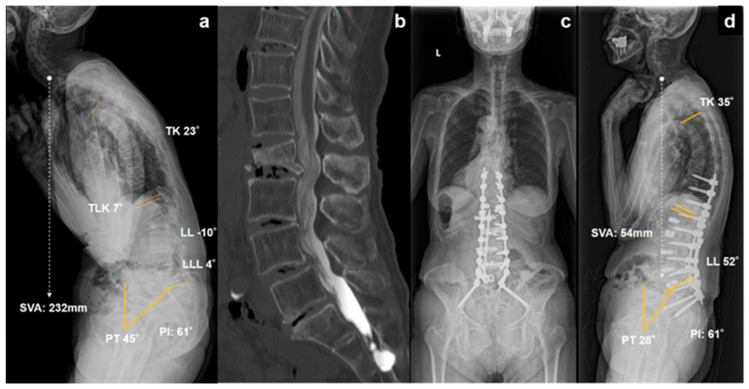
Representative case of long-fixation with pelvic fixation (Case 1). Preoperative standing lateral radiograph showing severe kyphotic deformity following an osteoporotic vertebral fracture (OVF) at L2 with decompensated sagittal malalignment (SVA 232 mm, LL −10°, LLL 4°) (a). Computed tomography demonstrated advanced degenerative changes in lower lumbar intervertebral discs (b). Postoperative anteroposterior (c) and lateral (d) standing radiographs after staged lateral lumbar interbody fusion and posterior long-segment fixation from T10 to the pelvis demonstrate satisfactory correction of global sagittal alignment.
